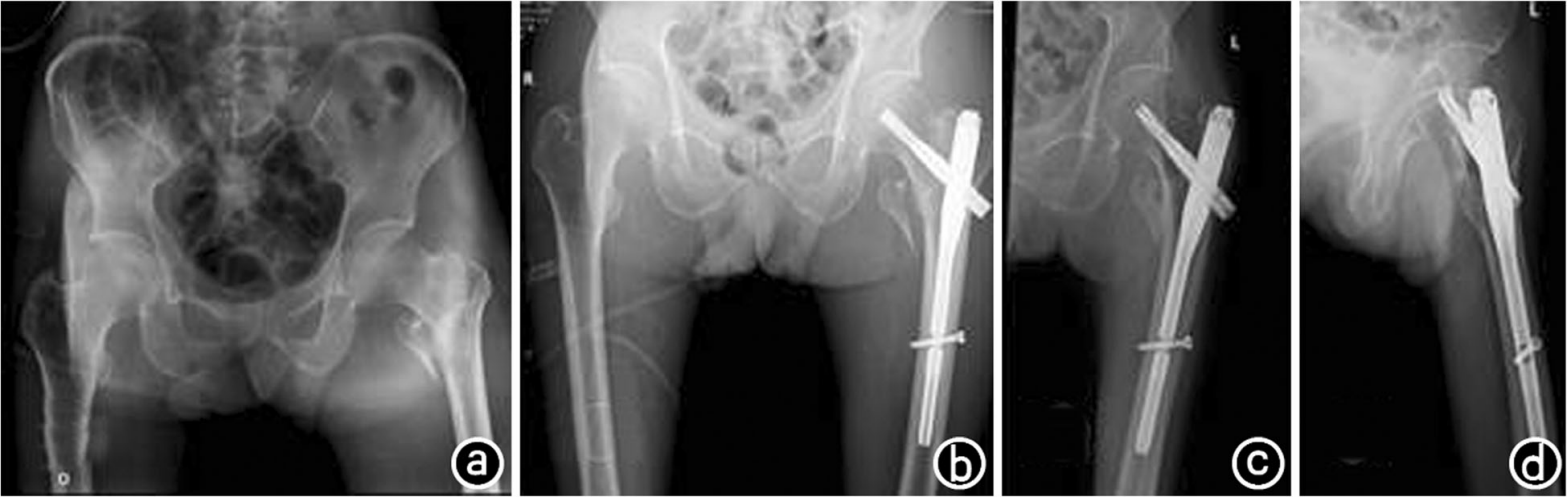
The patient was an 81-year-old male who accidentally fell on the ground while walking on March 25, 2012, causing left hip pain andlimited mobility. a. X-ray examination showed left comminuted intertrochanteric fracture and liberation of the great trochanter and lesser trochanter; b. Physical examination: adduction and internal rotation deformity was observed inhis left hip; left leg was approximately1.5 cm shorter than the right leg; percussion pain inhis large rotator and vertical percussion pain inhis limb. On March 27, 2012, he was treated with left intertrochanteric fracture fixation (PFNA) under nerve block anesthesia. Anteroposterior pelvis and lateral femoral examination after the operation showed good fracture alignment and satisfactory fixation; c, d. X-ray examination 2 years after the operation showed that the trochanteric fracture has healed without loosening or leakage of internal fixation
